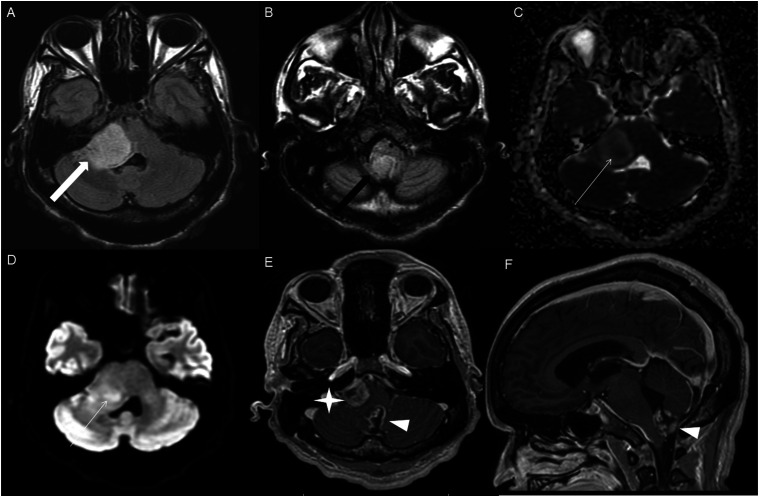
Axial FLAIR (A-B), DWI (C), ADC map (D), axial post-contrast T1 weighted image (E) and sagittal post-contrast T1 weighted image (F) of an NF1 patient show two concurrent FLAIR hyperintense tumors in different locations; the right middle cerebellar peduncle (A, thick white arrow), demonstrating heterogeneous post-contrast enhancement (E, star) and a focal area of diffusion restriction (C-D, thin white arrows) and the second tumor involving dorsal medulla and cerebellar tonsils (B, thick black arrow), with extension into the fourth ventricle, and showing peripheral, irregular enhancement (F, arrowhead).
